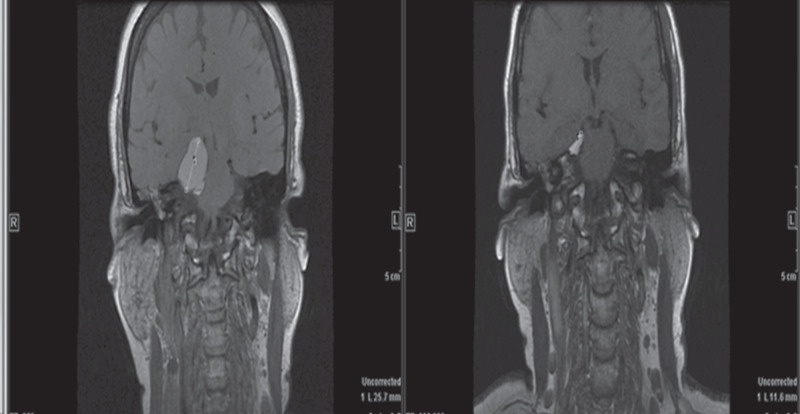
Intracranial response in a patient with adenoidcysitc carcinoma. Brain MRI in a patient with adenoid cystic salivary gland carcinoma showing significant shrinkage of intracranial tumour extension while receiving treatment with everolimus and lenalidomide.
